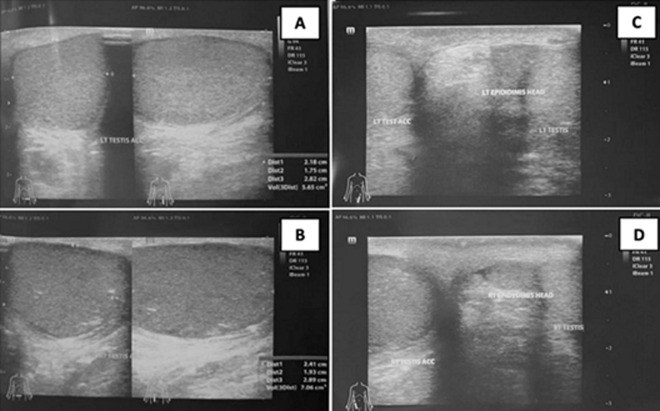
ultrasonography of scrotum showing: A) left and right; B) intrascrotal masses; C) the left and right; D) epididymides were within normal limits
